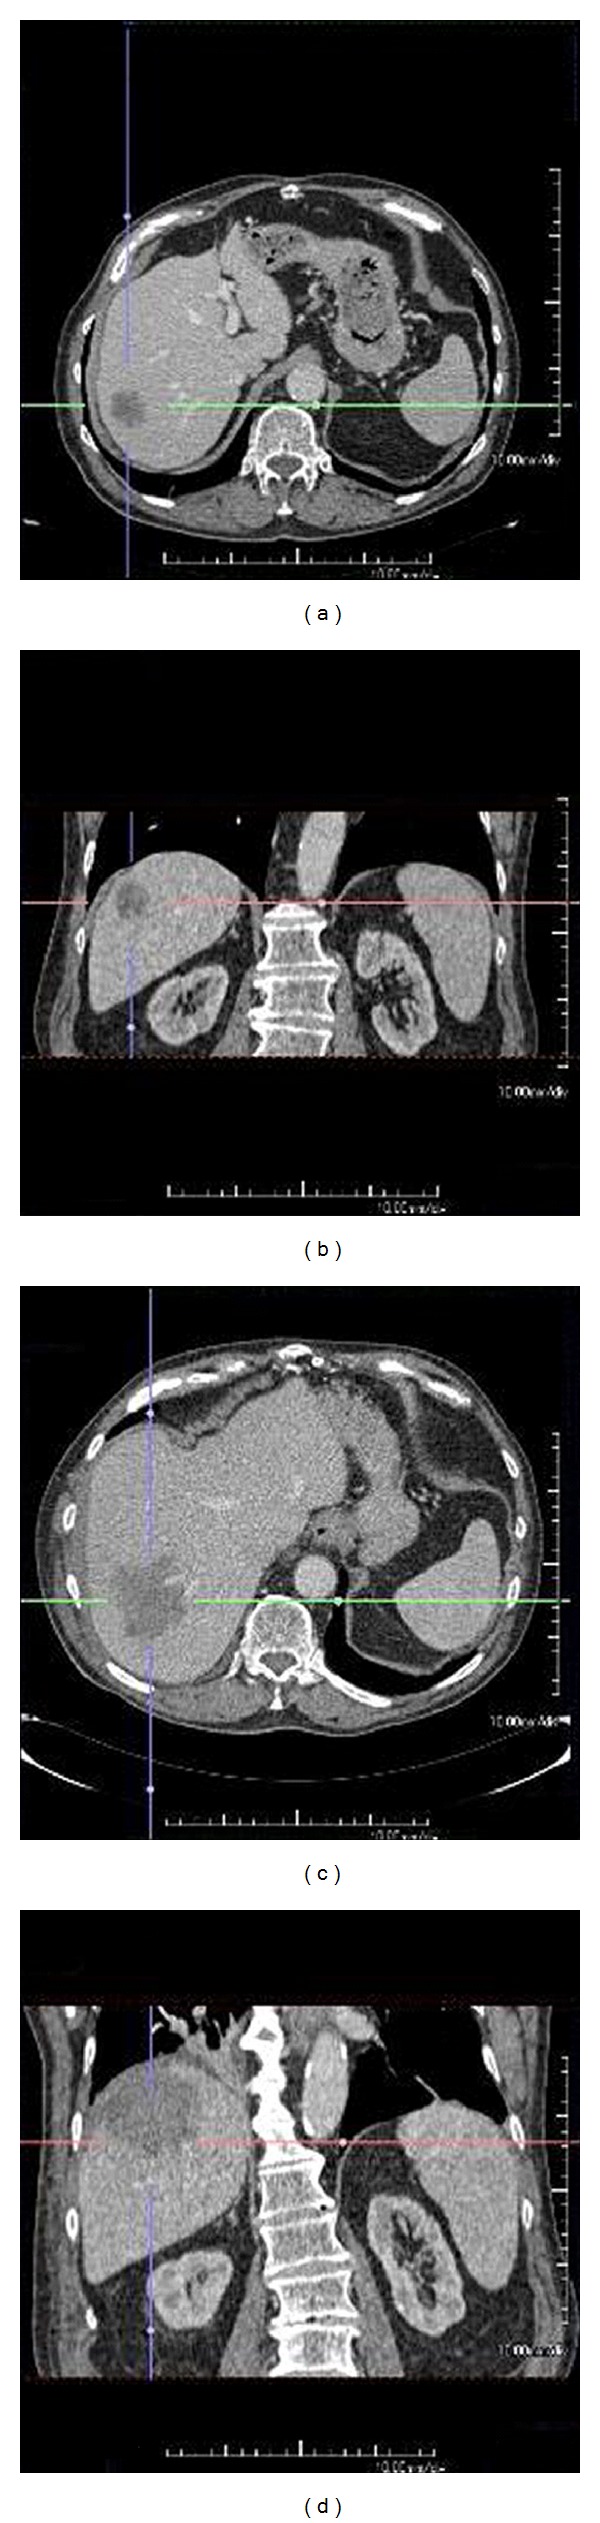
Similarity of Positioning Score-different (SiPS-diff). Example of nonidentical (discordant) pre-RFA CT-images and post-RFA CT-images, classified as Similarity of Positioning Score-different (SiPS-diff). The pre-RFA CT-scan ((a) axial, (b) coronal) and post-RFA CT-scan ((c) axial, (d) coronal) are not comparable.
